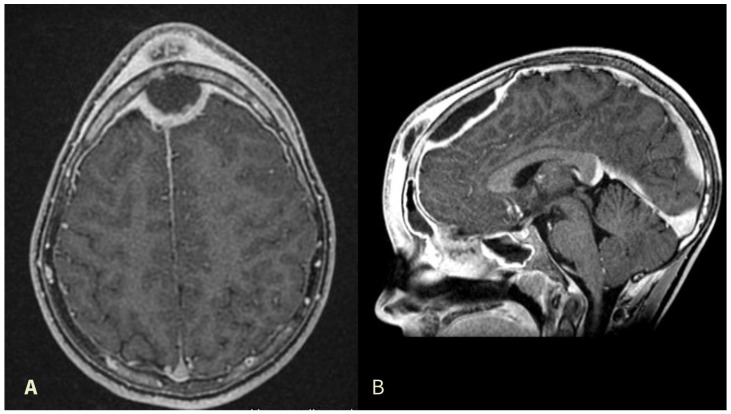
Preoperative contrast-enhanced MRI image revealing subcutaneous forehead swelling and a frontal epidural abscess in axial (A) and sagittal (B) views.
